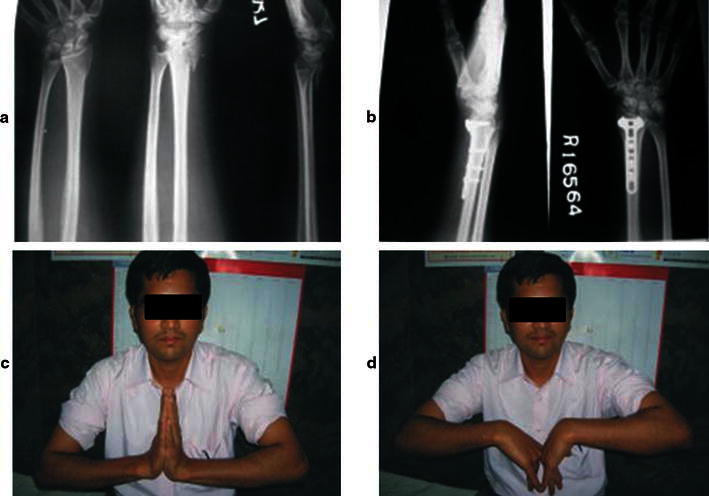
Pre- and postoperative radiographic image of the hand of a patient in volar plating (a, b). Clinical picture of a patient treated with volar plating after 1 year follow-up (c, d)
